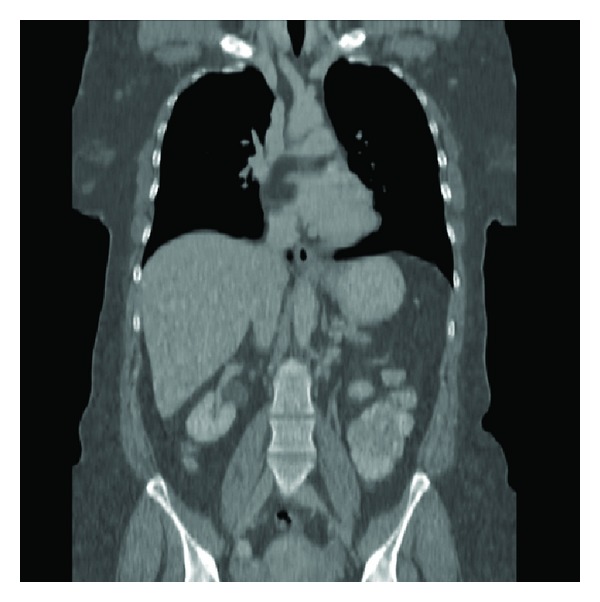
Coronal CT reconstruction shows how the superior vena cava tapers distally, as it is compressed by the lipoma.
